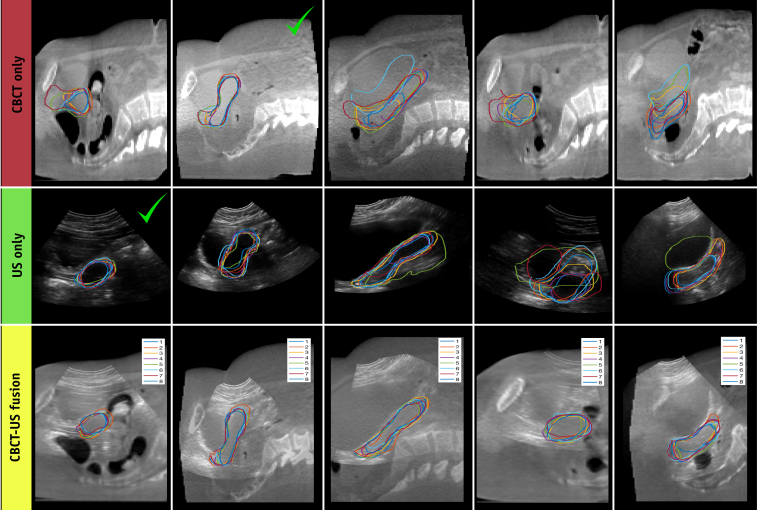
Comparison of interobserver contour agreement on cone beam computed tomography (CBCT) (pixel size [1, 1] mm), ultrasound (US) (pixel size [0.58, 0.58] mm), and CBCT-US fusion images (pixel size [0.58, 0.58] mm). Each observer's contour is denoted by the color given in the legend shown in the bottom row (Experienced observers = 1-3, New-to-US observers = 4-8). Columns 1-2: examples where the benefit of CBCT-US-fusion was mainly due to the increased probability of acquiring an excellent image from one modality (denoted by the green check marks). Columns 3-5: examples where CBCT and US images provided complementary information, which is why the interobserver agreement is relatively poor on both modalities individually but good on the CBCT-US fusion image. (A color version of this figure is available at https://doi.org/10.1016/j.ijrobp.2019.03.003.)
